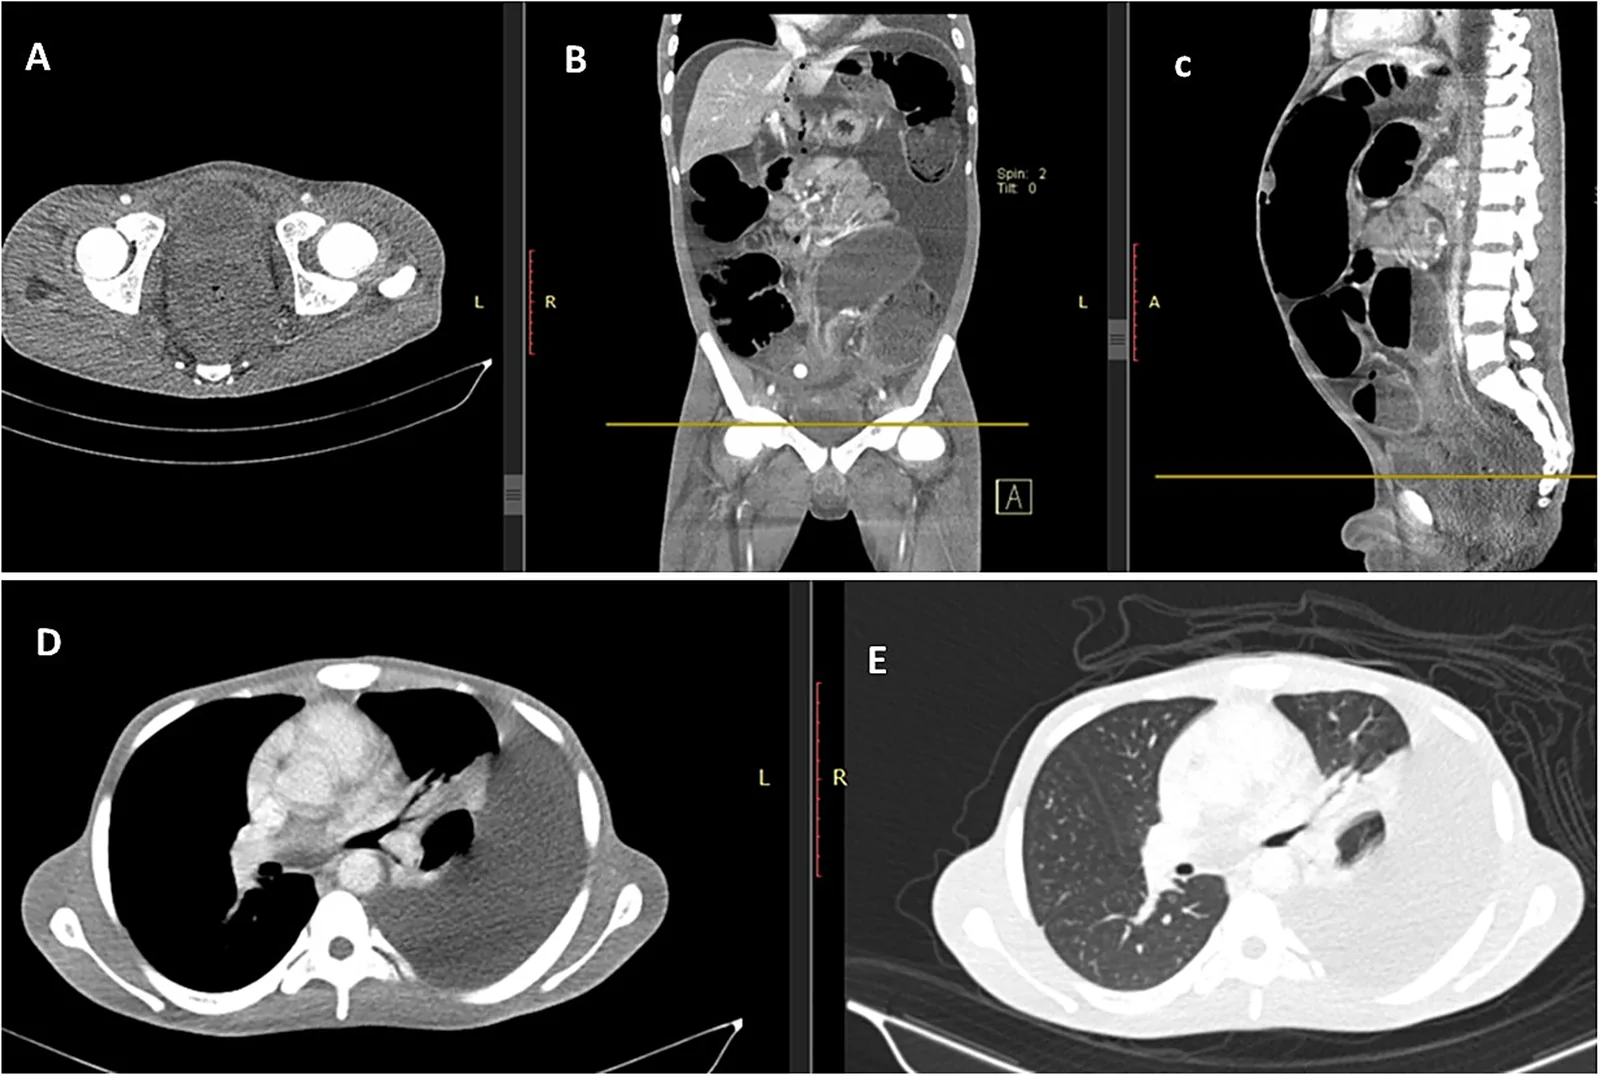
Contrasted abdominal-pelvic CT scan images; A (axial), B (coronal) and C (sagittal) planes shows marked recto-sigmoid stenosing symmetrical mural wall thickening of 4.2 and 16 cm in length with subsequent upstream large bowel obstruction. Small volume pneumoperitoneum suggesting tumor perforation, and moderate ascites noted. D (mediastinal window) and E (lung window) depicts severe left pleural effusion with left lower lobe compressive atelectasis. No metastatic pulmonary nodules.
